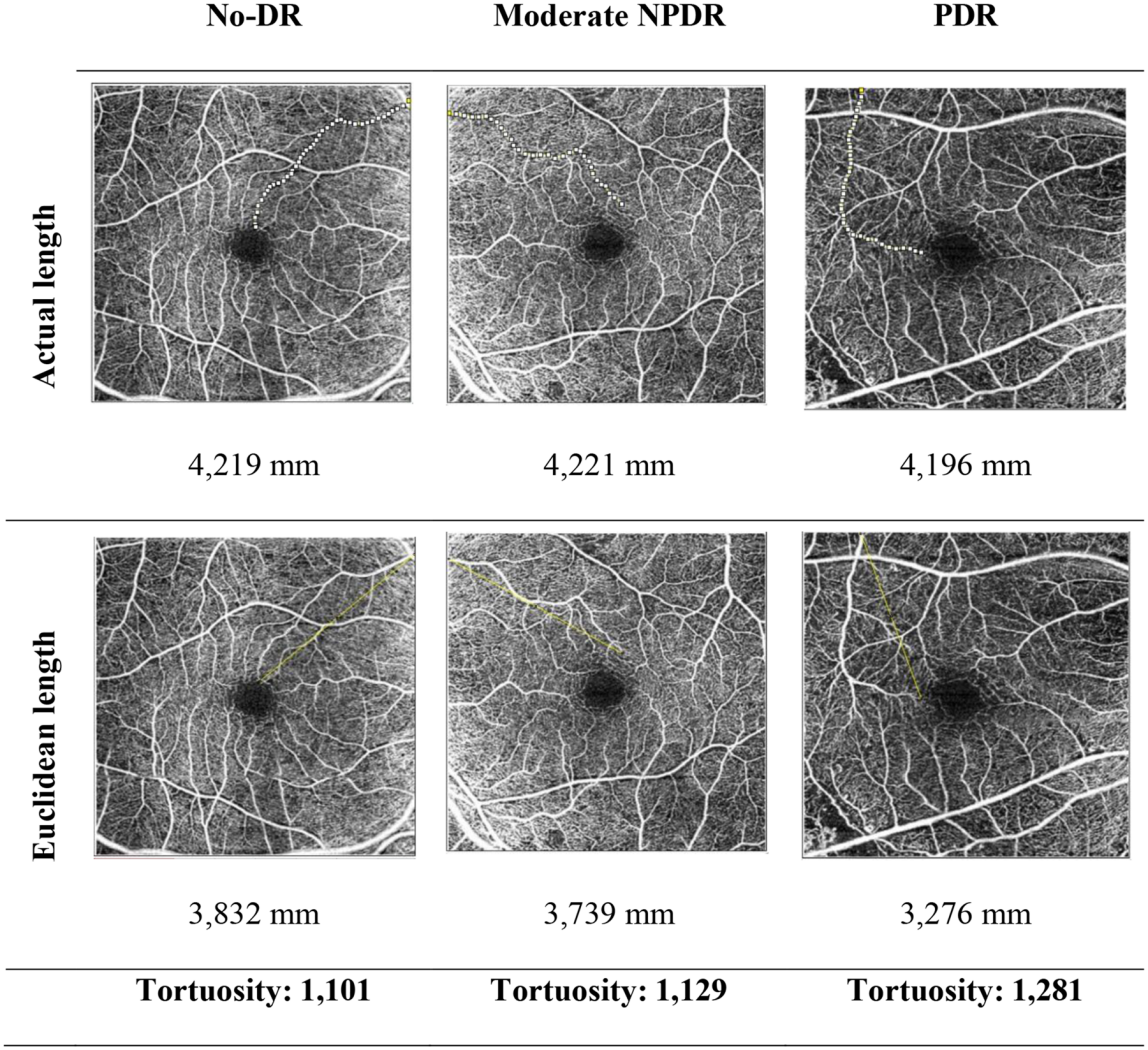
Representative image of tortuosity measurement from superficial capillary plexus OCT-A scan image. No DR, nondiabetic retinopathy; NPDR, non-proliferative diabetic retinopathy; PDR, proliferative diabetic retinopathy; OCT-A, ocular computed tomography-angiography
